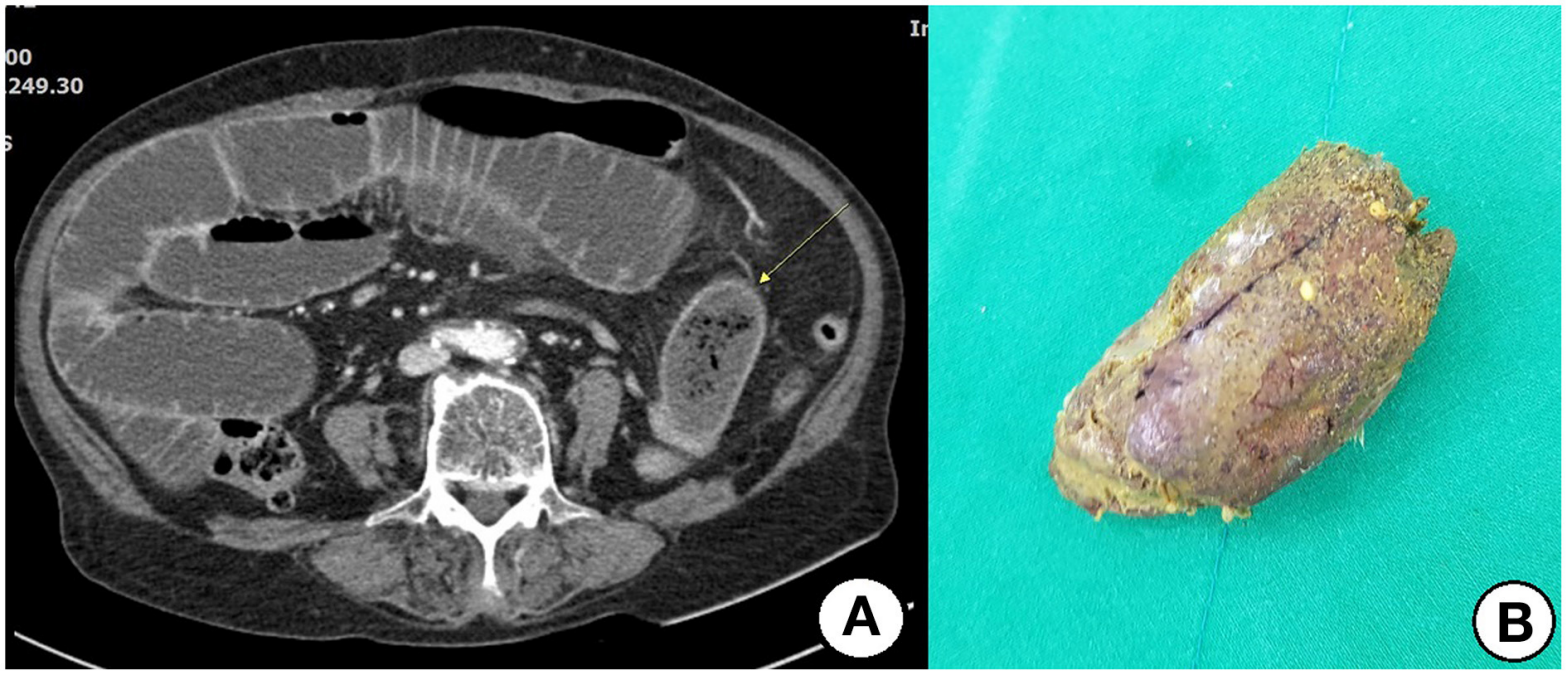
(A) Contrast-enhanced CT shows distended small bowel and an ovoid mass (arrow) with mottled gas pattern at the obstruction site. (B) Removed phytobezoar.
